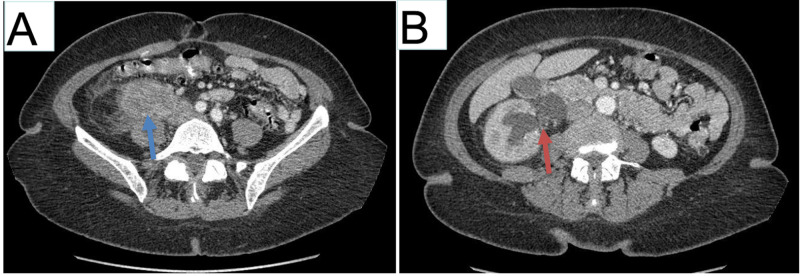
CT findings (A) Solid and cystic mass in the right retroperitoneum, measured 5.7 x 4.2 x 5.7cm^3^ in size (blue arrow). (B) Right hydronephrosis and proximal and mid-right hydroureter (red arrow) due to external compression effect of the mass.
